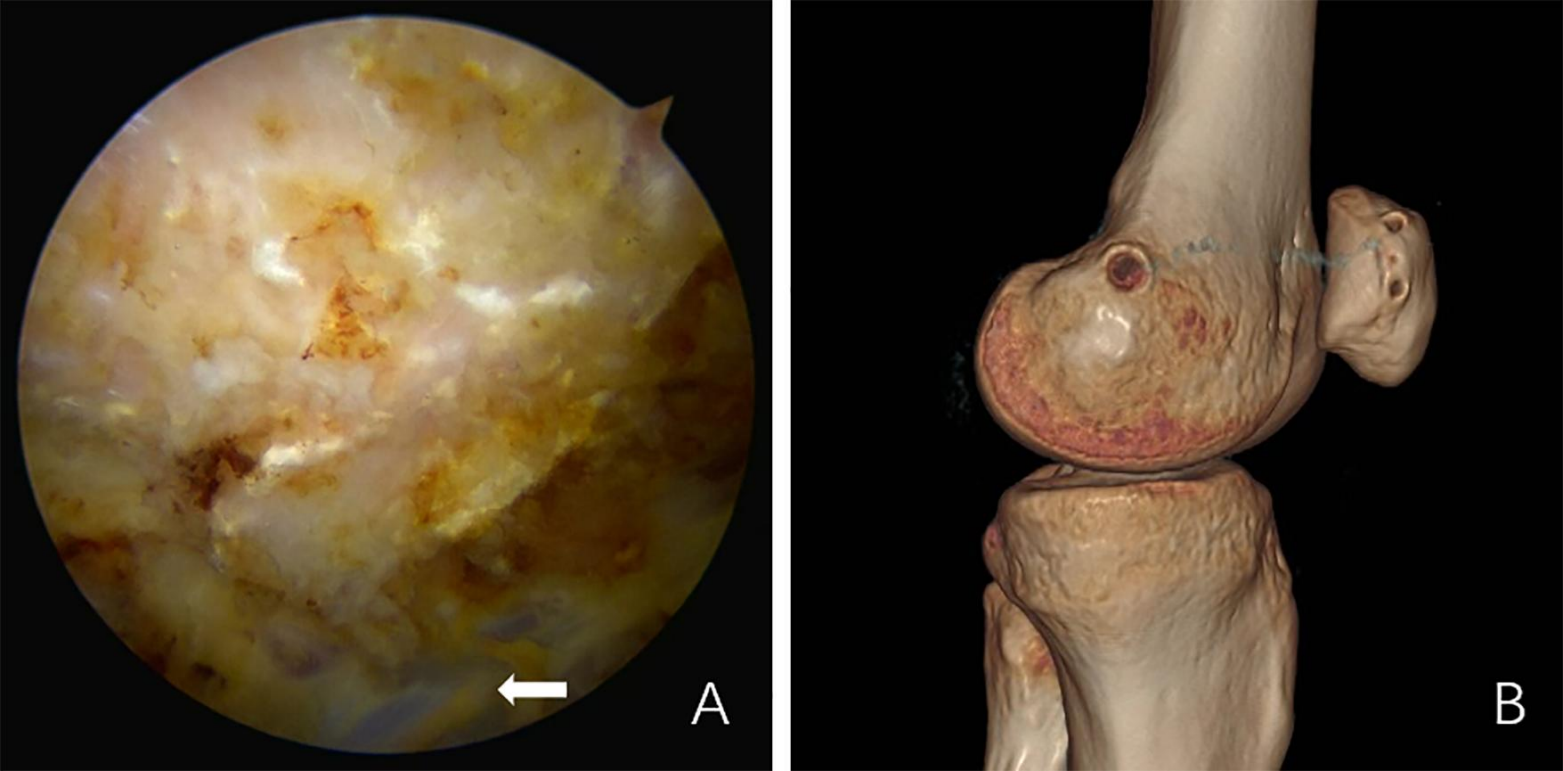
MPFL reconstruction. (A) Intraoperative arthroscopic anchor fixation of the semitendinosus tendon (arrow). (B) Postoperative CT 3D reconstruction shows the position of the anchors in the medial groove of the patella and the medial femoral condyle.
